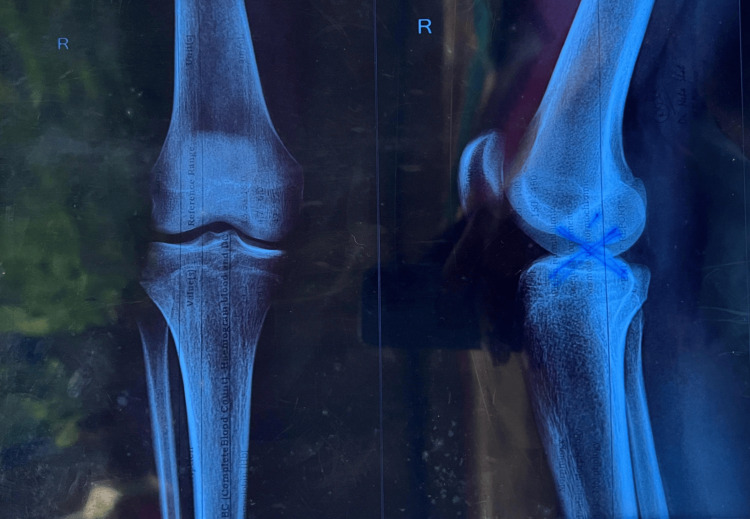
Knee radiograph of a patient taken just before the surgery.
